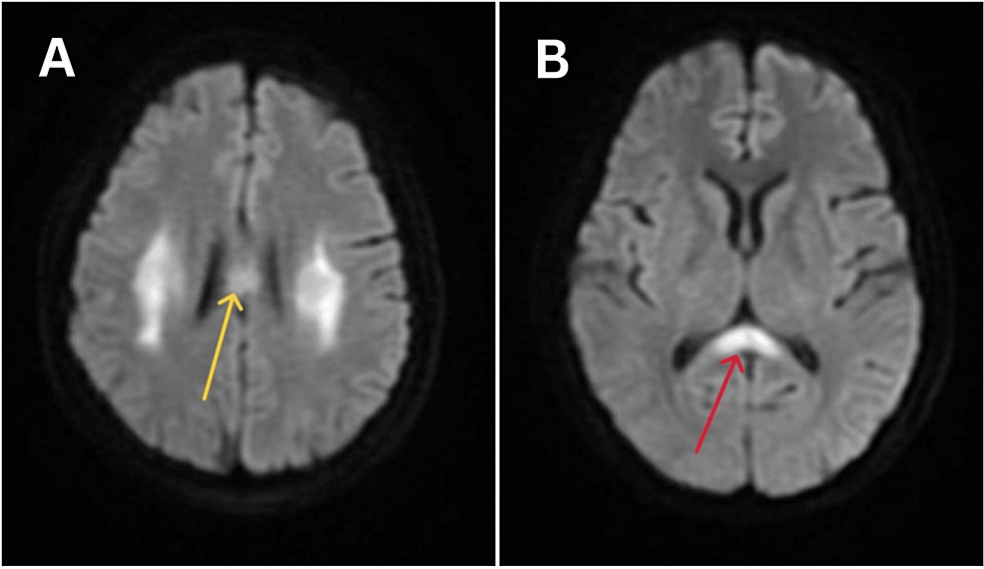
Magnetic resonance imaging of the brain of the patient suggestive of diffusion weighted-imaging sequences of the axial section showing A) hyperintensities in the body of the corpus callosum (yellow arrows) B) hyperintensities in parietal lobes (red arrows)
